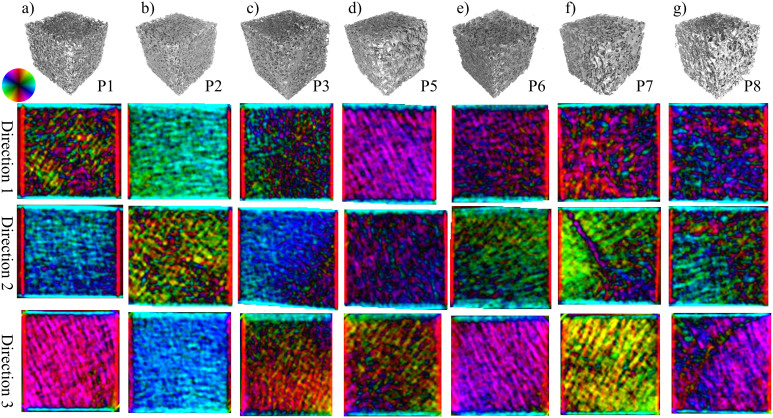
Shown is a *μ*CT rendering and the results of three calculations of the XVR signal, based on *μ*CT data. The samples and directions correspond to those shown in figure 4. The direction and anisotropy of the main structural orientation is encoded in the same way as for the XVR results in fig. 3.
